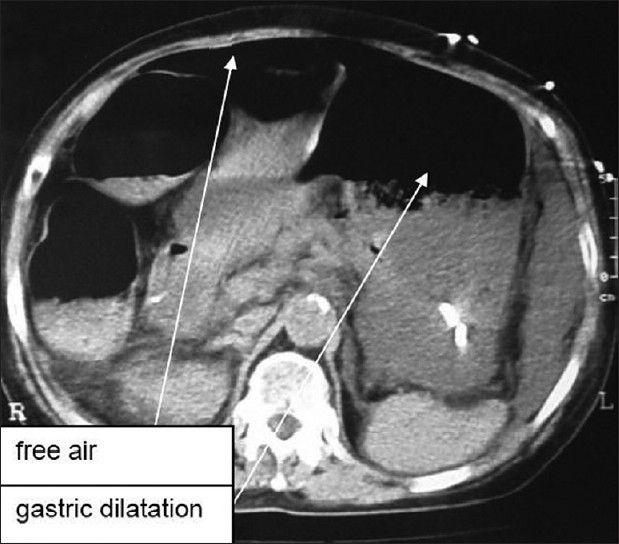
Computed tomography scan showing gastric dilatation and intraperitoneal free air
